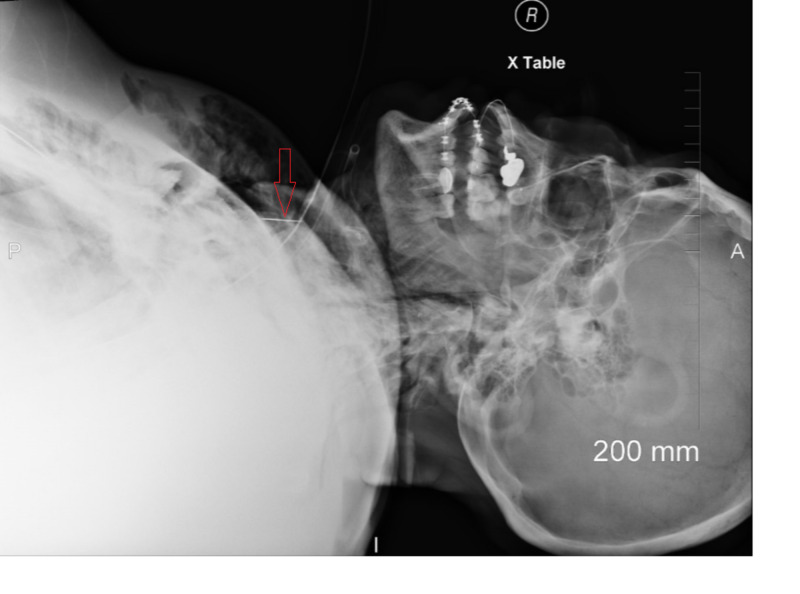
Chest X-ray lateral view showing scalpel blade (labeled)
